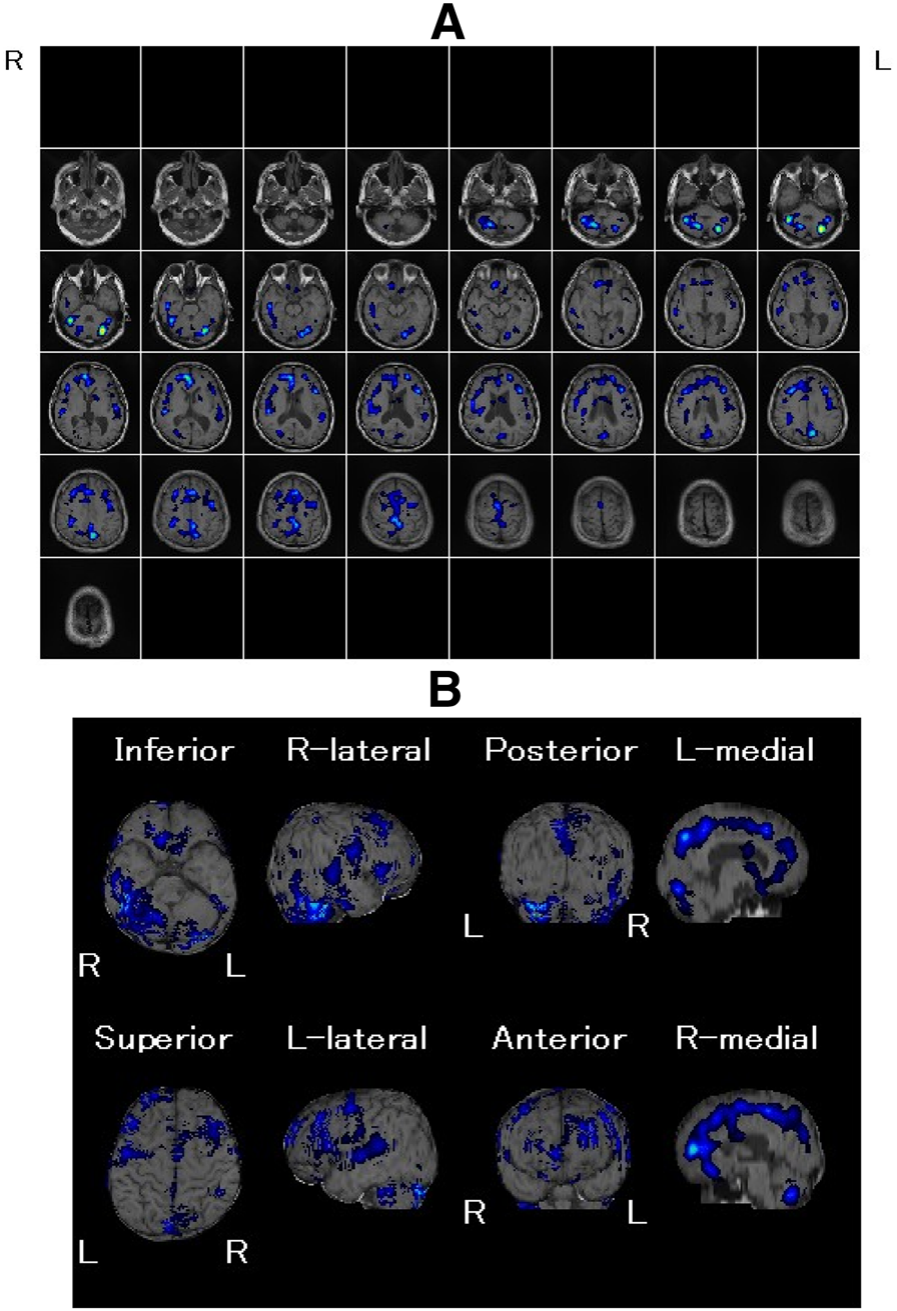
eZIS from ictal - interictal Tc-99 m ECD-SPECT (A), (B). This figure shows hyperperfused areas compared with the standard database. Hyperperfusion is shown as a blue to yellow area in the frontal cortex. This figure is typical of the “frontal type” of eZIS pattern.
